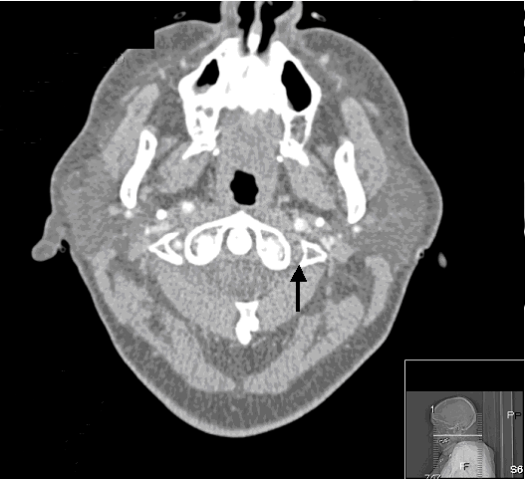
Computed Tomography Angiogram of patient C. The black arrow indicates a dissection of the left vertrebrate aretery as a result of deceleration trauma.
